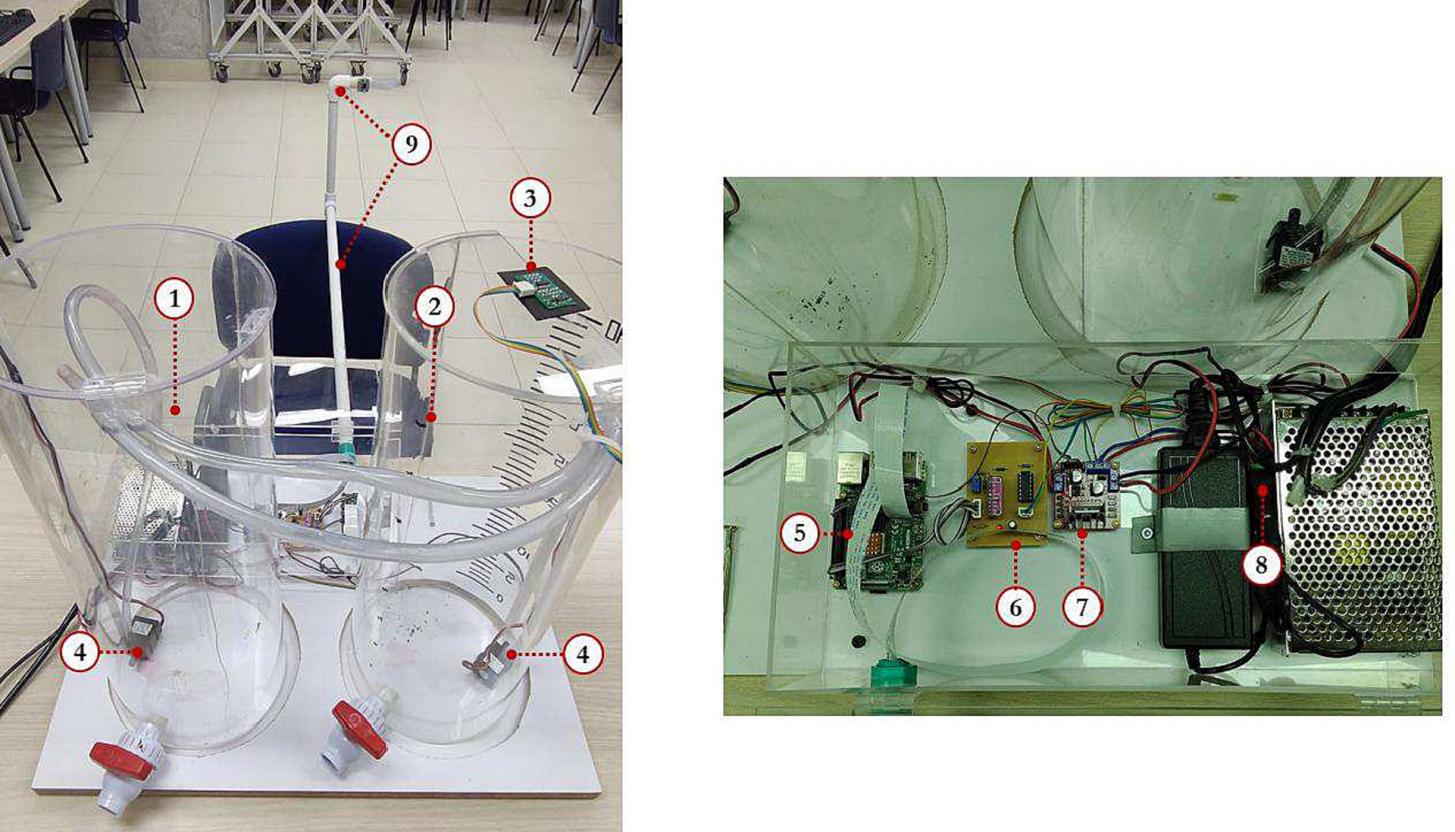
Overall hardware appearance of *RaspyControl Lab*. 1. Reservoir tank (T2), 2. Main control tank (T1), 3. Analog ultrasonic sensor, 4. 12 V motor pumps, 5. Raspberry Pi 4, 6. Signal conditioning circuit with ADC (ADS1115), 7. L298 motor pump driver, 8. 12 V and 5 V Power supply sources, 9. Raspberry pi camera with PVC pipe support.
